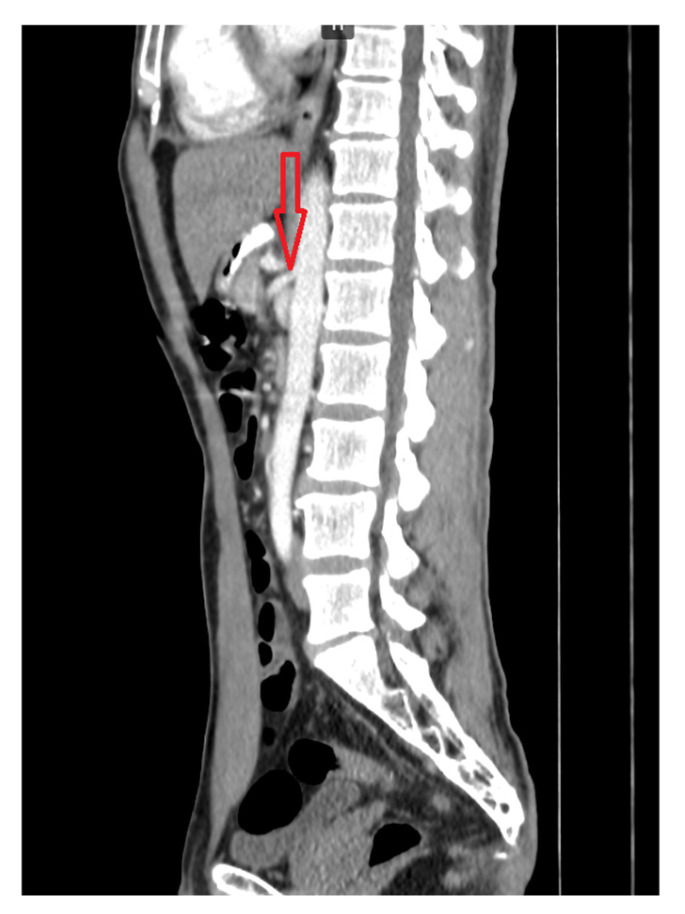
Contrast-enhanced multislice computed tomography (MSCT) showing compression of the horizontal (third) part of the duodenum between the superior mesenteric artery and the abdominal aorta (arrow).
